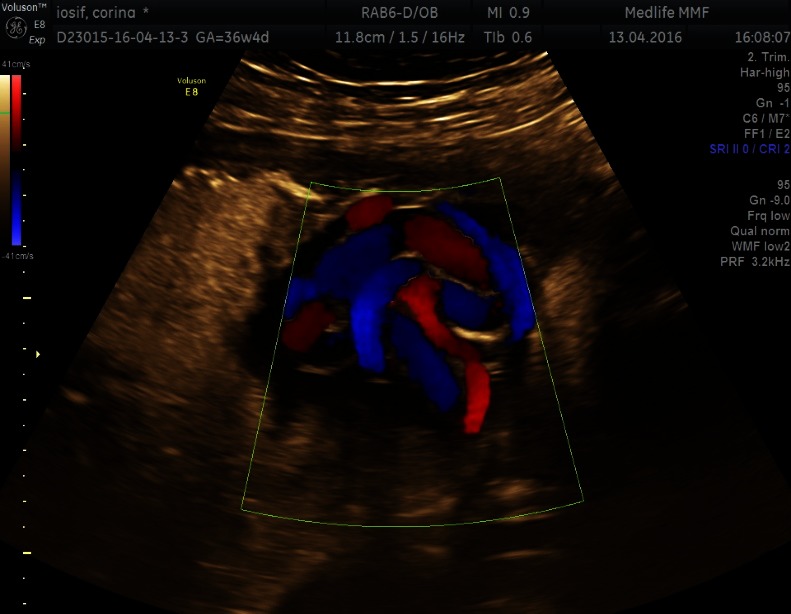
False umbilical cord knot on color Doppler
